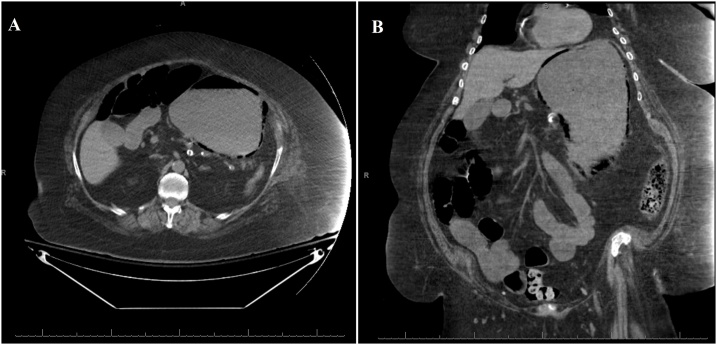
Non-contrast computed tomography scan of the abdomen revealed gastric distention and gastric pneumatosis throughout the wall of the stomach.
